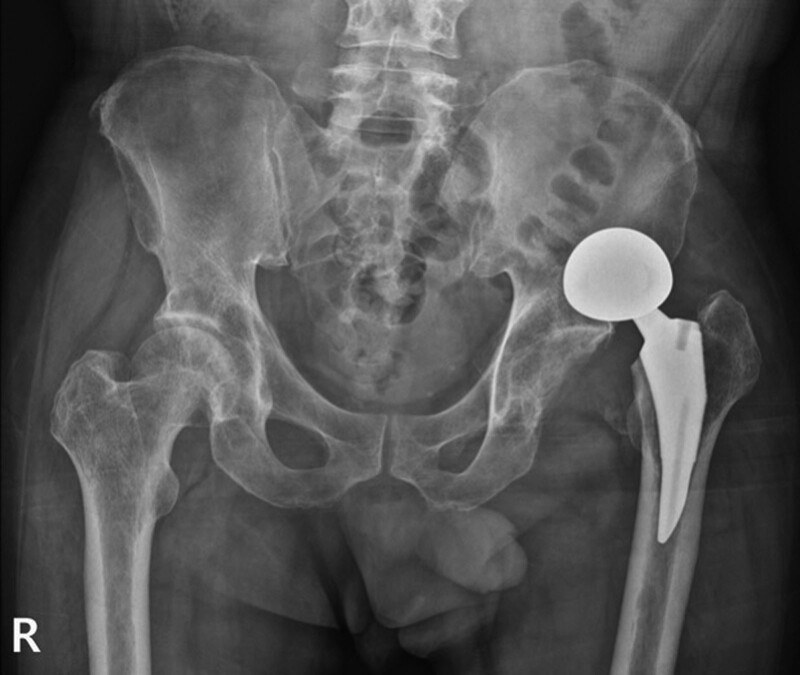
Anteroposterior radiograph demonstrating the third dislocation in hip hemiarthroplasty. The femoral head component appeared as an elliptical shape and not “O”-shaped.
